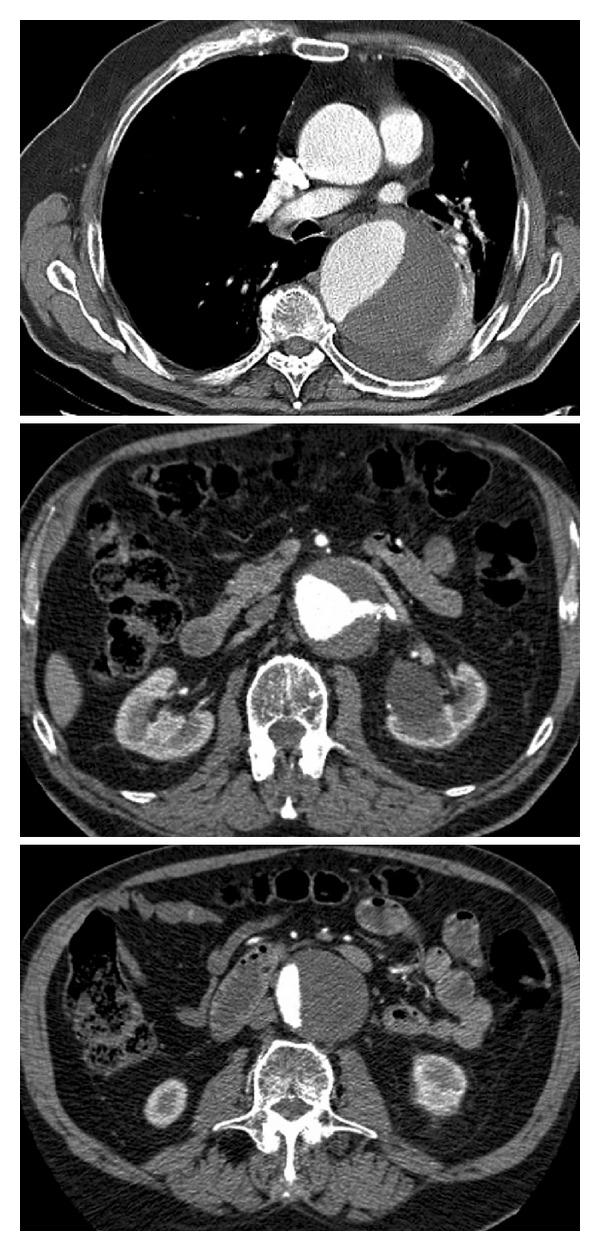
Computed tomography sections at the level of the descending aorta (upper panel), at the level of the left renal ostium (middle panel), and at the level of the infrarenal aorta (lower panel).
